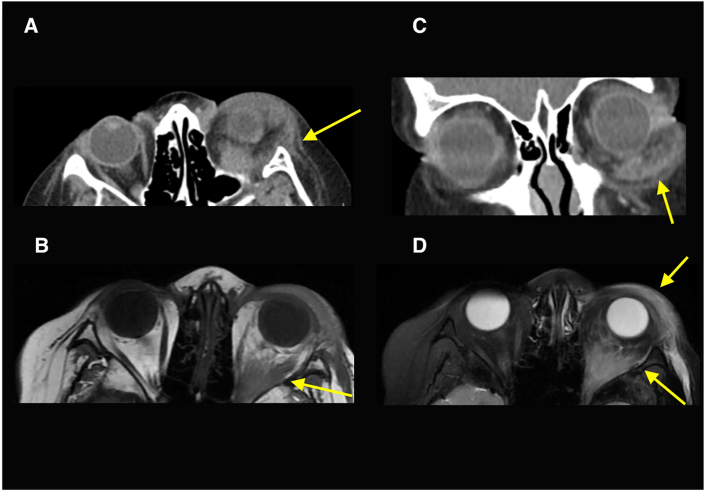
Axial and coronal CT orbits with contrast demonstrating periorbital soft tissue swelling with evidence of post-septal extension, consistent with orbital cellulitis (A,B). MRI orbits obtained the next day demonstrates similar orbital and periorbital inflammatory changes, but also notes thickening and enhancement of the inferior and lateral rectus muscles (C,D).
